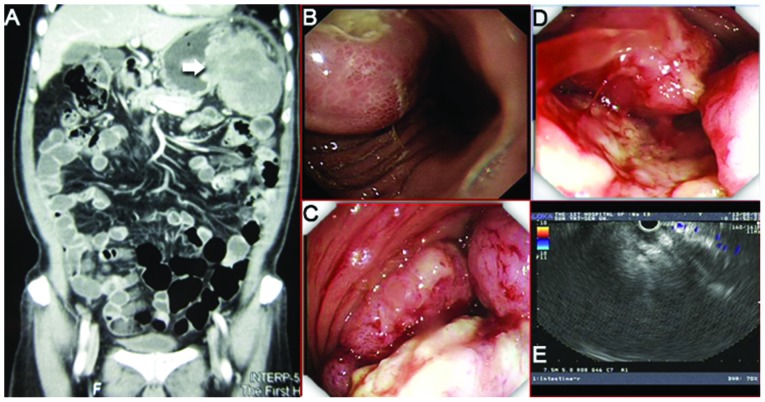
Radiographic findings of the patient following admission to The First Affiliated Hospital of Sun Yat-Sen University (Guangzhou, Guangdong, China). The mass was located under the diaphragm, with good demarcation and full invasion of all layers of the stomach and colon. (A) Coronary computed tomography scan. (B) Gastric endoscopy. (C and D) Colonoscopy. (E) Endoscopic ultrasonography. A total bowel obstruction was observed due to tumor invasion.
